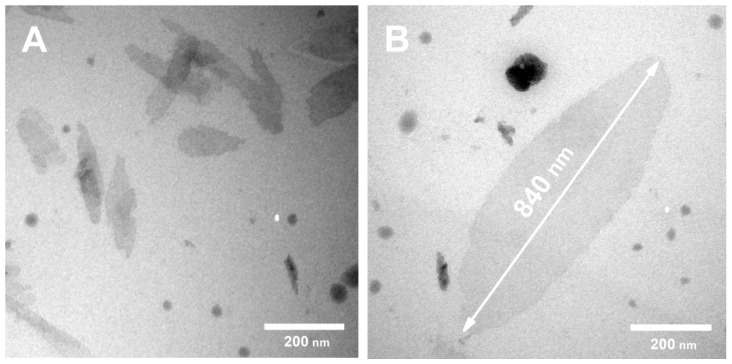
TEM-images of graphene particles, stabilized with PVP (A) and Plu (B). The particles of different sizes were obtained depending on the type of stabilizer and the duration of ultrasonic treatment. The duration of ultrasonic treatment of Plu-stabilized dispersions is 1–2 h, in case of PVP-stabilized it was 2–4 h.
